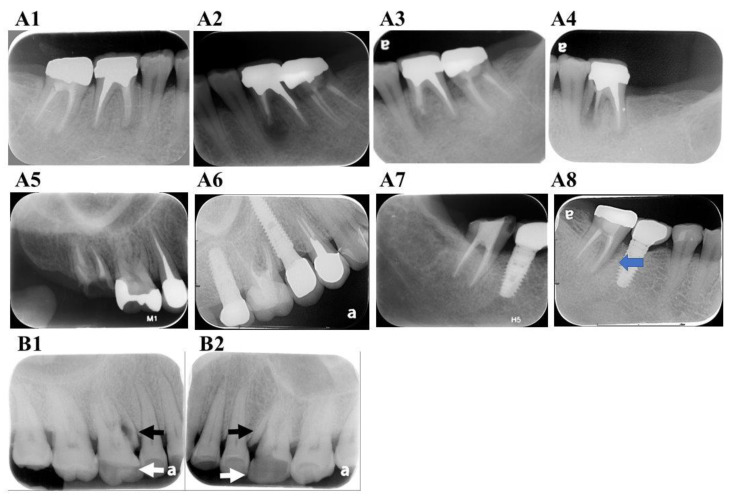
Clinical cases of VRF. (A1) A 31-year-old female patient showed 46 clinical symptoms even after endodontic retreatment and crown fabrication in January 2006. A VRF in the mesial root with deep pocket was noted after surgical exploration. (A2) In the same patient, a periapical radiograph of 36 was taken immediately after apical surgery of the mesial root in December 2011. Apical radiolucency was noted. (A3) Complete healing of apical lesion over the mesial root of 36 in May 2012. (A4) Sinus tract formation and a deep periodontal pocket with VRF of the mesial root of 36 was found in October 2013. (A5) Radiograph picture of 15, 16 and 17 in June 2016. Endodontic retreatment of 17 was completed. Endodontic treatment and crown procedure performed several years ago in the local dental clinic. (A6) Radiograph of the same region in November 2020; 15 and 17 were extracted due to VRFs and replaced with implants during this period. (A7) Endodontic treatment of 47 was completed in May 2013. (A8) VRF of 47 with deep pocket and radiolucency (blue arrow) around the whole mesial root of 47 in November 2020; (B) VRFs (black arrows) in non-endodontically treated teeth usually present along with an attrited occlusal surface (white arrows). (B1) 16 and (B2) 26 in the same patient.
